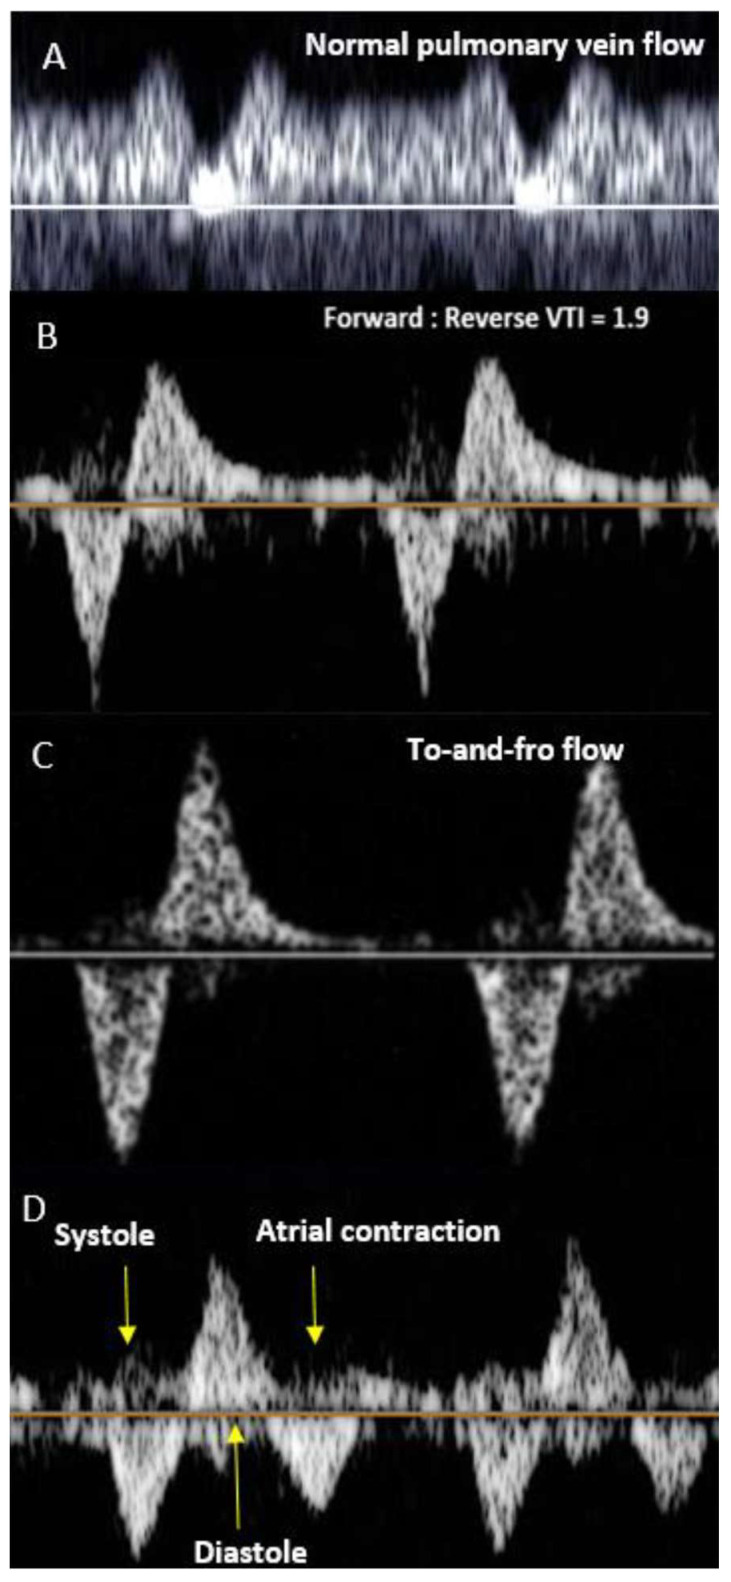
(A) Normal pulmonary vein flow pattern with phasic flow. No flow reversal seen in diastole. (B) Pulmonary vein Doppler flow pattern in HLHS with a severely restrictive atrial septum as evidenced by a forward to reversed velocity-time integral of < 3 mm. (C) Pulmonary vein flow pattern typically seen in the setting of an intact atrial septum, showing to-and-fro flow. (D) Double reversal sign seen in severely restrictive or intact atrial septum and severe mitral valve regurgitation.
